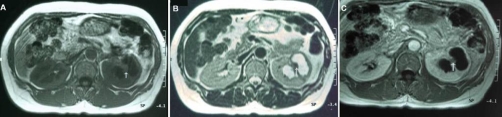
Transverse T1-weighted (A), T2-weighted (B) and Gadolinium (Gd) -enhanced T1-weighted (C) images are presented. Left kidney has cystic mass lesion at upper pole, which is composed of thinned parenchyma and water signal component. The abnormal renal parenchyma is demonstrated with low signal intensity on both T1 and T2-weighted images. A small-sized parenchymal nodule (arrow) is also depicted isointense and hypointense on T1 and T2-weighted images, respectively, when compared to the normal renal parenchyma. The abnormal parenchyma is heterogeneously enhanced and the nodule is not enhancing after Gd administration.
